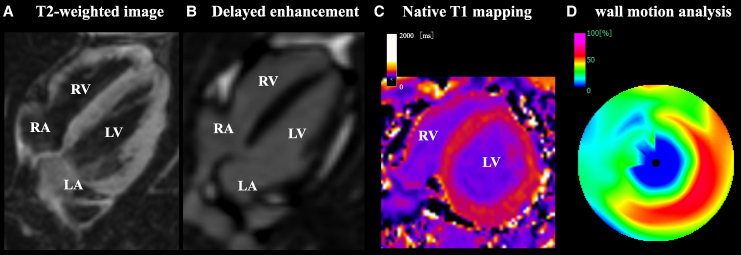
Gadolinium-enhanced cardiac magnetic resonance imaging of a 38-year-old woman. T2-weighted image (*A*), delayed gadolinium enhancement (*B*), native T1-mapping (*C*), and wall motion analysis (*D*) are presented, respectively. Findings suggestive of myocarditis, such as myocardial oedema, pericardial effusion, or late gadolinium enhancement indicative of myocardial scarring, were absent. Consistent with the echocardiography findings, regional wall motion abnormalities confined to the apical segments are noted.
